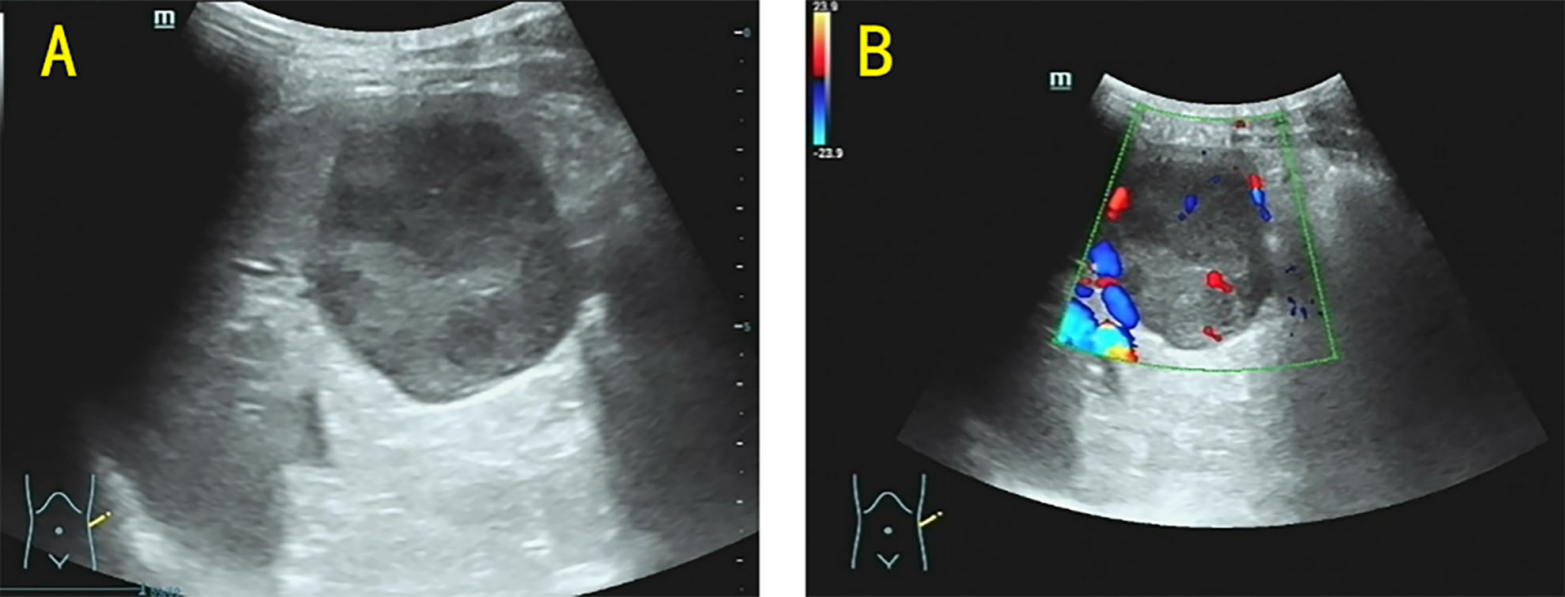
(A) Ultrasound revealed a hypoechoic mass of approximately 48 × 48 mm in size in the lower pole of the spleen, with well-defined borders. (B) The color Doppler revealed that blood flow signals were visible within the mass.
